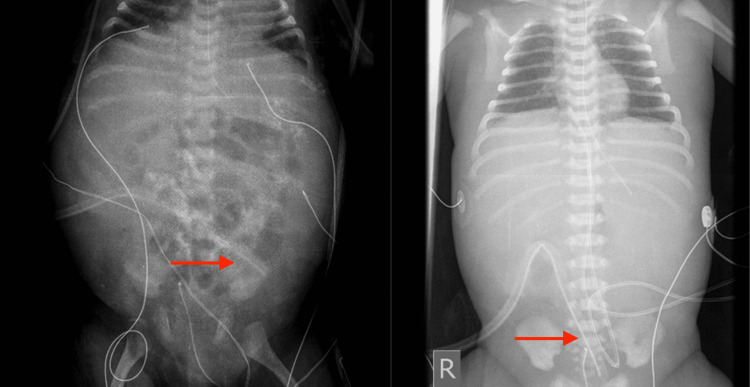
Abdominal X-ray on the 16th and the 73rd day of life showing the positioned Tenckhoff catheter. Red arrows indicate the catheter.
